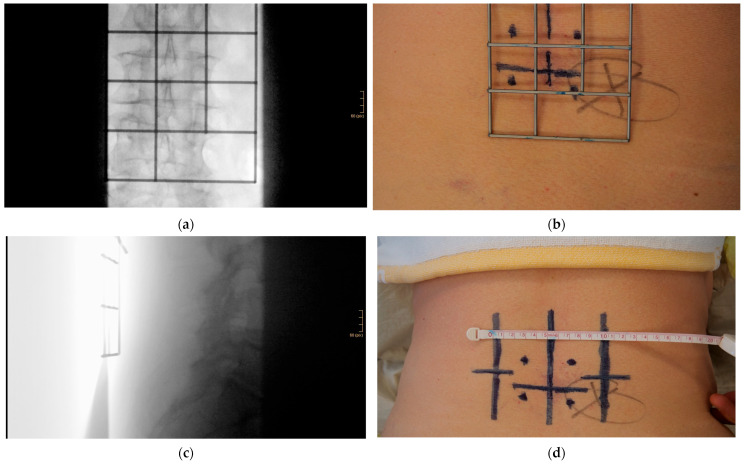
(a) A metal template is used as a localization tool to identify the correct segment as well as the involved pedicles and intervertebral discs with one single ap radiographic image. (b) The midline, pedicles, and intervertebral disc are marked. (c) One additional lateral fluoroscopic image depicts the plane of the paramedian transverse skin incisions, usually at about the level of the upper pedicle. (d) The laterality of the skin incision is marked by two longitudinal lines (here, 5 cm paramedian). The required metal grid had already been removed in the photo. In this study, the Sextant II system (Medtronic) was primarily used. For other minimally invasive screw–rod systems with rod insertion through the same skin incision as the pedicle screws, a longitudinal skin incision may be considered meaningful. Note the constant application of beam collimation in each fluoroscopic image.
